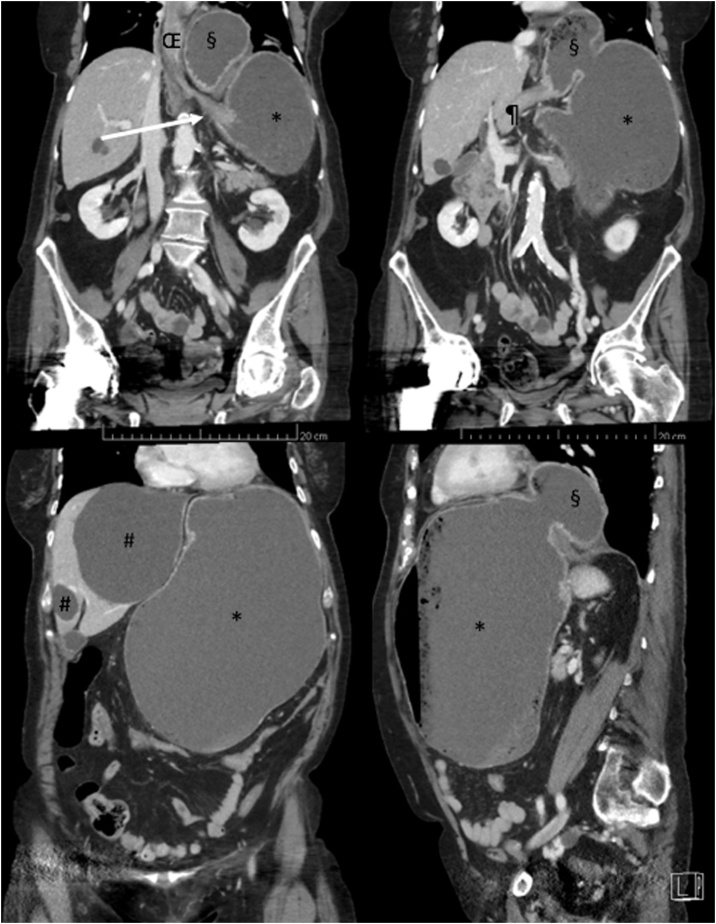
Abdominal CT (coronal view on the upper left, upper right, lower left; and sagittal view on the lower right) at initial presentation showing the mesenteroaxial gastric volvulus with the antrum (§) above the gastroesophageal junction (white arrow), exerting a mass effect on the distal esophagus (Œ). Other symbols refer to duodenum (¶), biliary cysts (#) and distended stomach (*).
